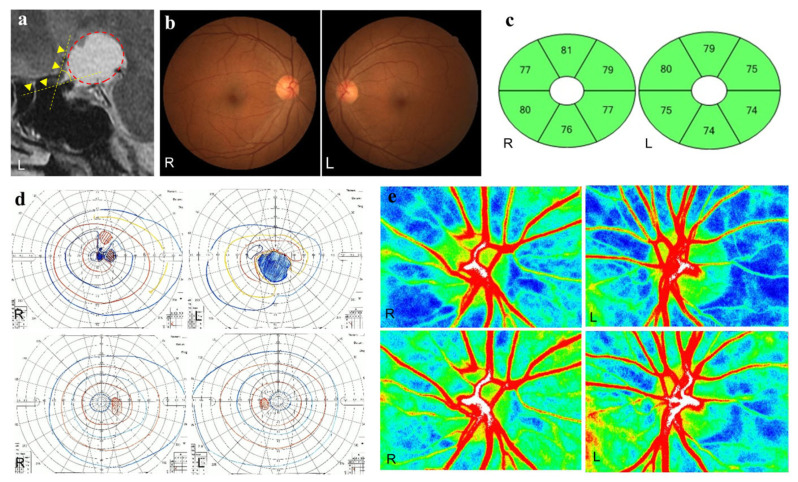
A representative case of craniopharyngioma. A 57-year-old woman has optic nerve bending in her left eye caused by craniopharyngioma. Preoperative and postoperative best-corrected visual acuities (BCVAs; logarithm of the minimum angle of resolution units) are 0.30 and −0.08 in the right eye and 2.0 and −0.08 in the left eye, respectively. (a) Sagittal T2-weighted magnetic resonance images before surgery. The optic nerve-canal bending angle (ONCBA) (yellow dotted lines) is formed by the optic nerve in the optic canal and the optic nerve in the intracranial subarachnoid space at the optic canal’s exit. The yellow arrowheads indicated the optic nerve. A circle surrounding red dots indicates the tumor. The ONCBAs of this case are 10° in the right eye and 63° in the left eye. (b) Color fundus photograph of both eyes showing the normal appearance at the preoperative visit. (c) The thickness of the ganglion cell layer (GCL) + inner plexiform layer (IPL) in both eyes after tumor resection is within the normal range. (d) Goldmann perimetry before and after tumor resection. A central scotoma is detected in both eyes before tumor resection. The preoperative SVFIS score for the left eye is lower than that for the right eye (4 points for the right eye; 12 points for the left eye) (upper panels). After tumor resection, the central scotoma has disappeared in both eyes, and the SVFIS score is 0 for both eyes (lower panels). (e) Laser speckle flowgraphy (LSFG) images of the optic nerve head (ONH) before and after tumor resection. The preoperative optic nerve head-mean blur rate (OHN-MBR) of the overall region (MA), vessel region (MV), and tissue region (MT) are 23.1, 46.3 and 11.9 in the right eye, and 22.2, 37.2 and 9.3 in the left eye, respectively (upper panels). Postoperative MA, MV and MT for the right eye are 27.9, 54.9 and 12.0; for the left eye, they are 29.6, 55.0 and 11.3, respectively (bottom panels).
